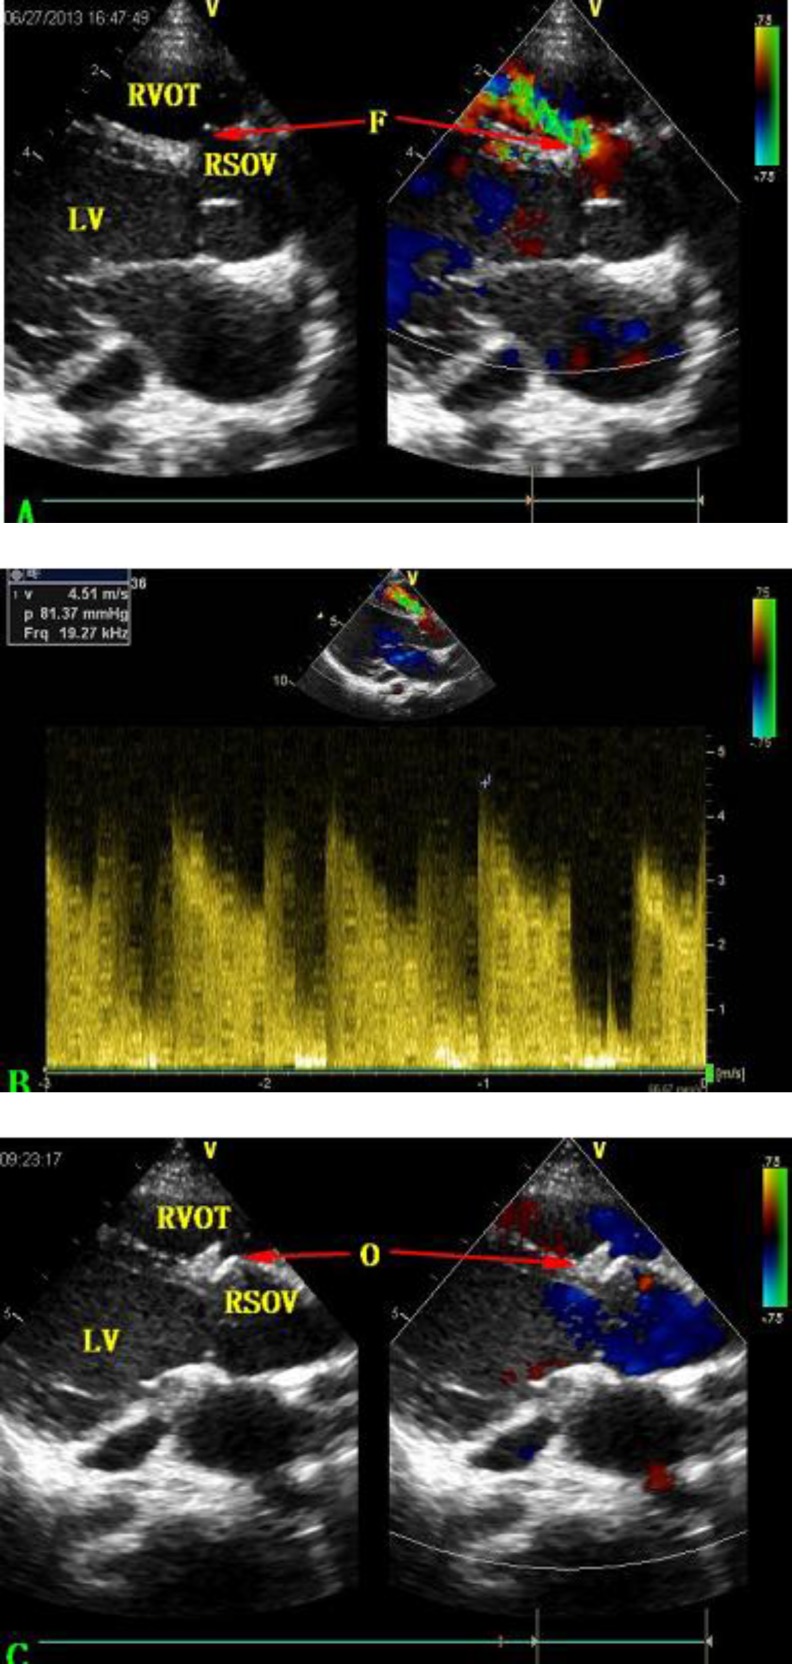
A: Transthoracic echocardiography showing the fistula of RSOV to RV. B: Color Doppler showing a continuous shunt from RSOV to RV with high velocity (4.5 m/s). C: Transthoracic echocardiography revealing satisfactory position of the occluder, with neither residual shunt nor aortic regurgitation
